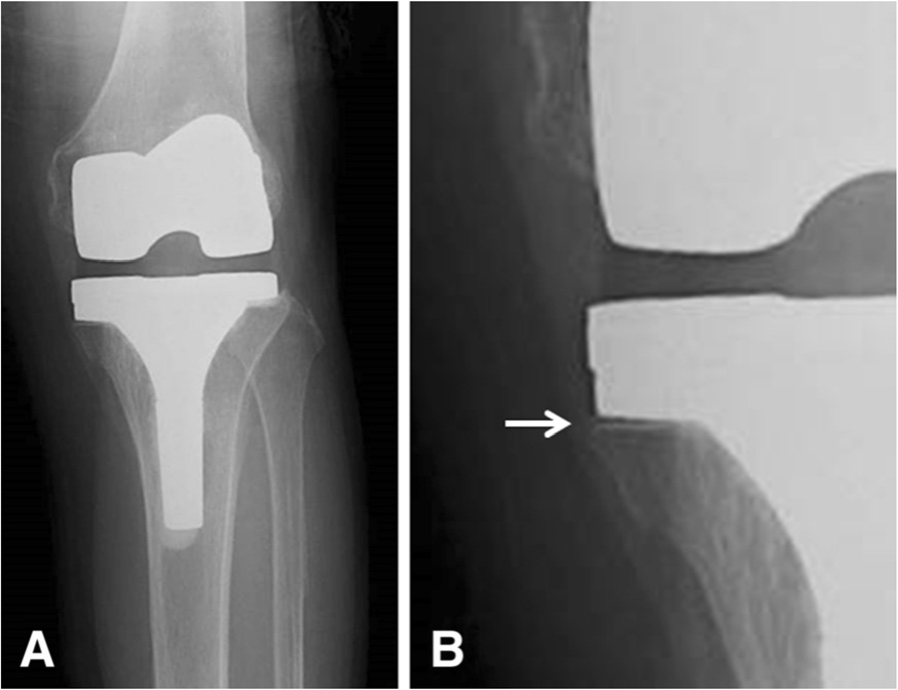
One-year postoperative anteroposterior radiographs revealing the radiolucent line beneath the metal augmentation (arrow) in an 85-year-old woman. (A) Overall view of radiograph. (B) Magnification of the medial tibia.
